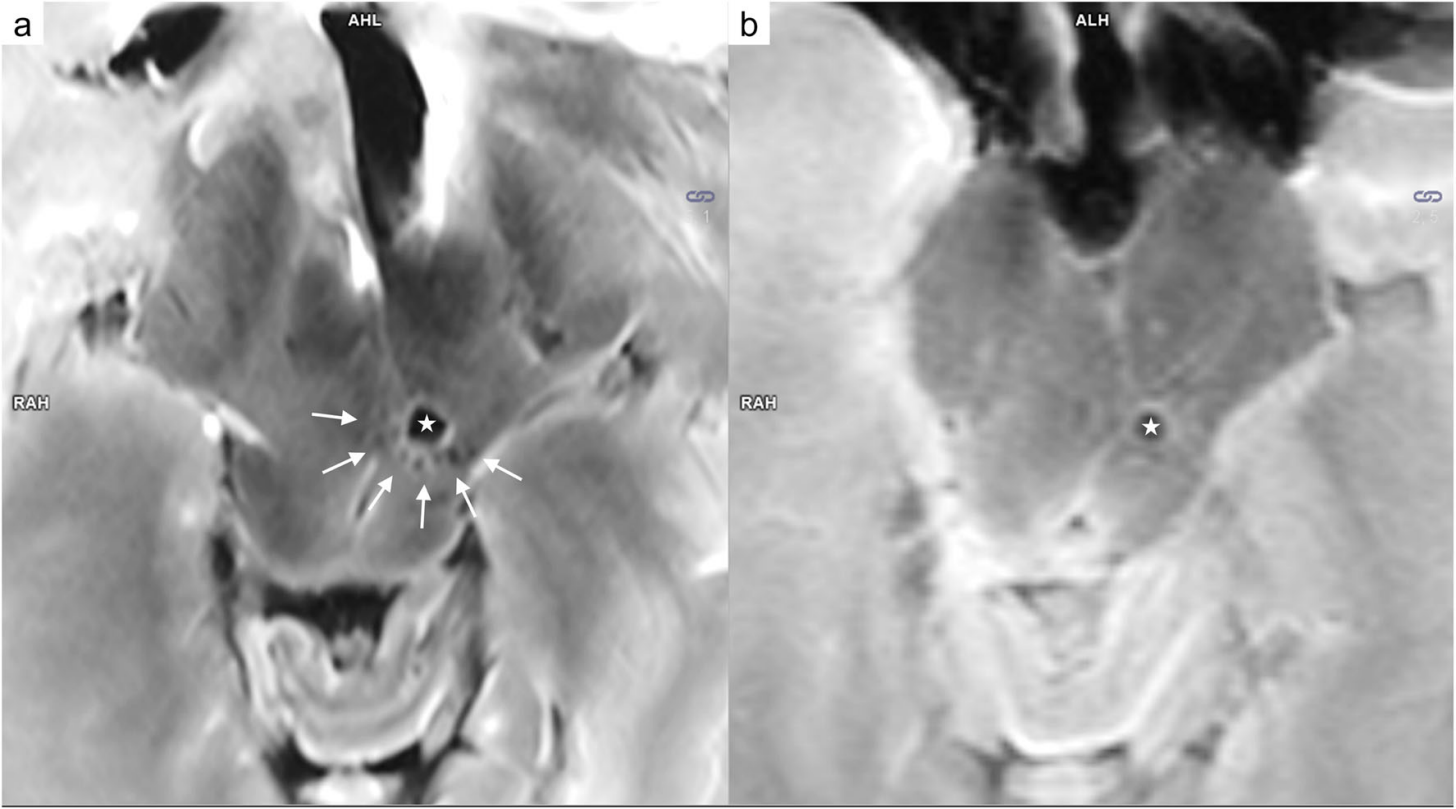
Comparison of 7 T T_2_-SPACE images (a) and 3 T T_2_-TSE-SPAIR images (b) obtained in case 3 in a transversal view. Only 7 T MRI enabled identification of several microbleeds (arrows: tiny dark/hypo intense dots) surrounding the wound tract (asterisks)
